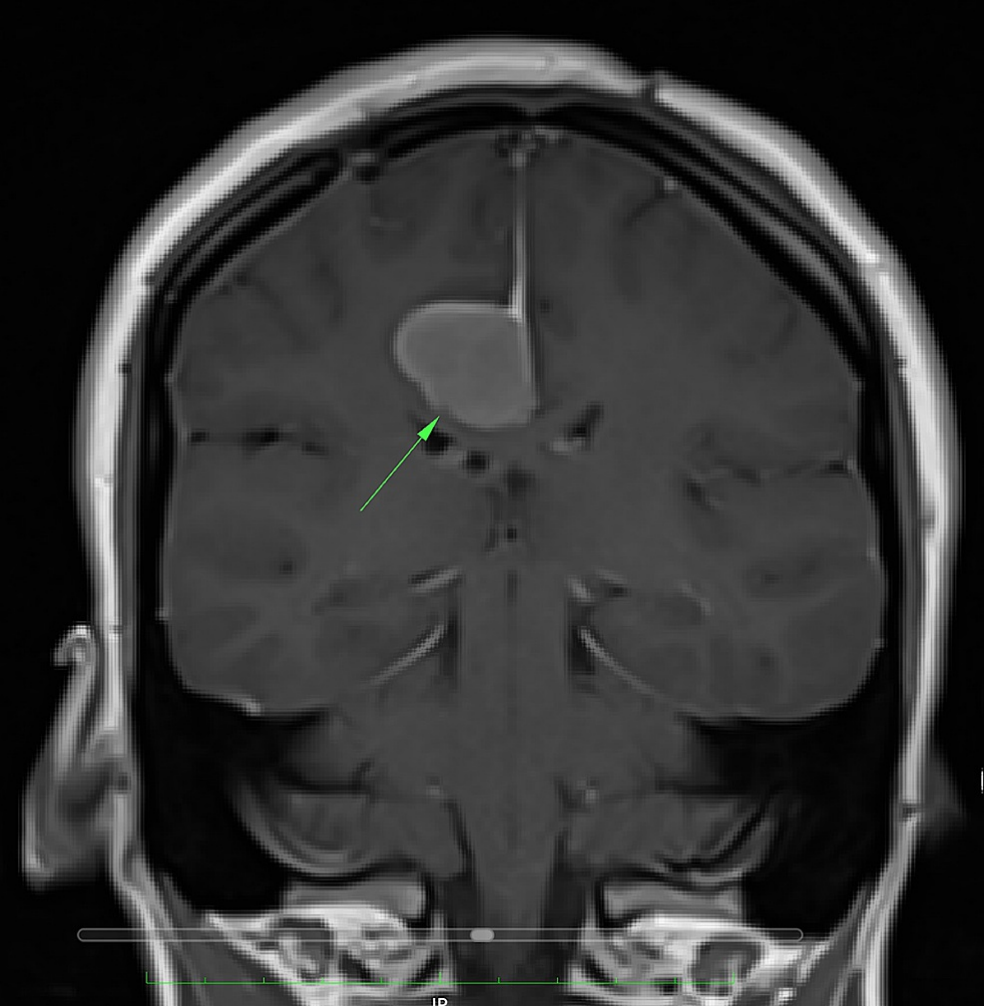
Coronal T1 MRI with contrast showing the space-occupying lesion marked by the arrow. MRI: magnetic resonance imaging
